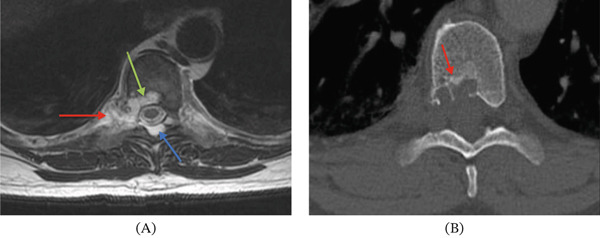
These axial images of the thoracic spine show the dominant T8–T9 lesion, which was diagnosed as chordoma after needle biopsy. (A) Panel A shows a lobulated T2‐hyperintense mass with well‐defined margins involving bone (green arrow), with paraspinal (red arrow) and epidural (blue arrow) extension on MRI. (B) Panel B shows a lytic appearance on CT with sclerotic borders (red arrow). These features are radiologically consistent with chordoma.
